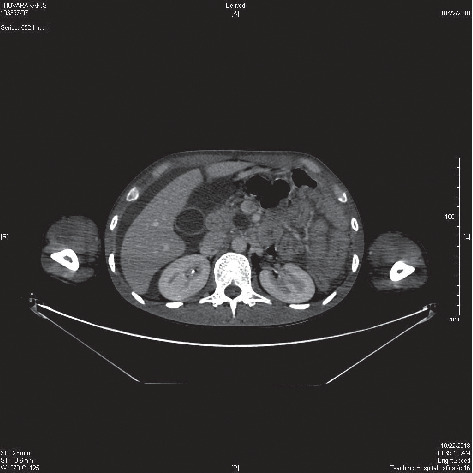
The contrast-enhanced computed tomography of abdomen shows two cystic collections suggestive of pseudo cysts in pancreas.
